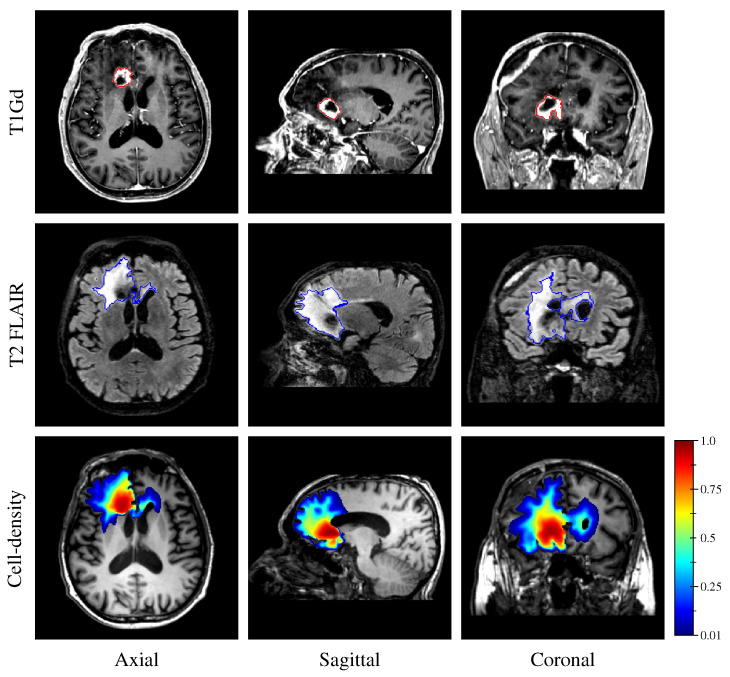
T1Gd image (1st row), T2 FLAIR image (2nd row), and estimated three-dimensional tumor cell-density distribution using the first network (3rd row) for an IDH-wildtype glioblastoma patient in axial (1st column), sagittal (2nd column), and coronal (3rd column) planes. The contours of the segmented enhancing core and peritumor vasogenic edema are superimposed in red on the T1Gd (1st row) and in blue on the T2 FLAIR (2nd row) images, respectively.
